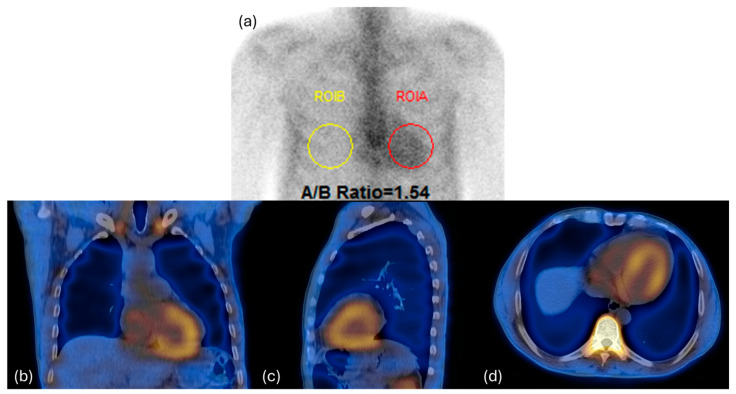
Whole-body and SPECT-CT acquisition of a 55-year-old patient presenting cardiac ATTR, Perugini Grade 2: (a) heart-to-contralateral ratio; (b) coronal SPECT-CT; (c) sagittal SPECT-CT; and (d) axial SPECT-CT.
